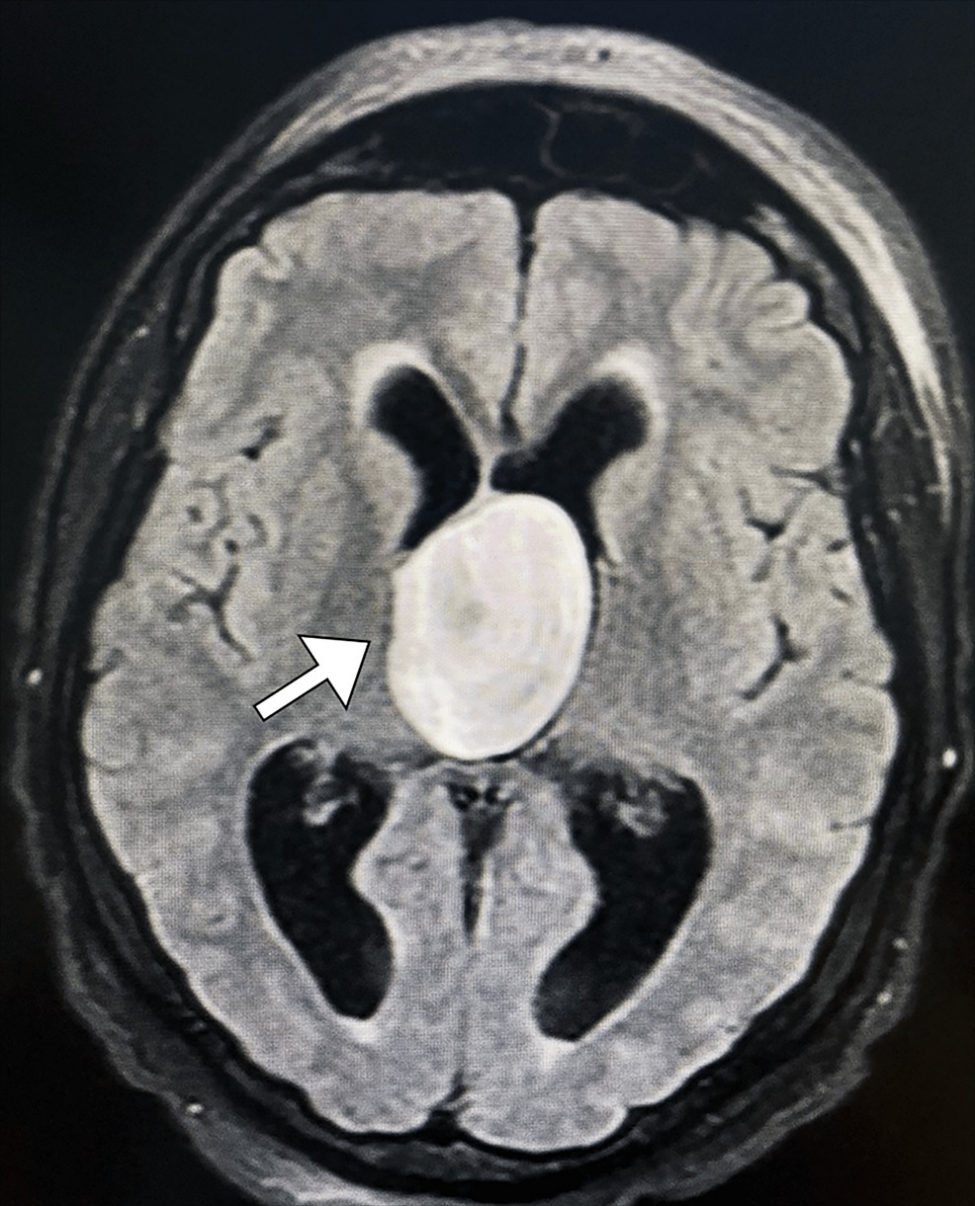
Axial FLAIR MRI image of the brain displaying a large, heterogeneous mass arising from the roof of the third ventricle, resulting in obstructive hydrocephalus FLAIR: fluid-attenuated inversion recovery
